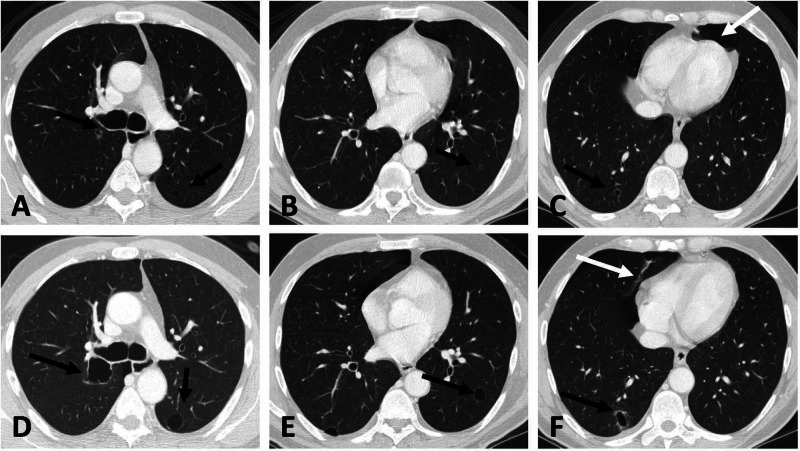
Enhanced CT at six-month follow-up (lung window) The thin wall cysts demonstrate enlargement in size exceeding 50% in volume and increase in wall thickness exceeding 3 mm in thickness, becoming cavitary in nature (images A, B, and C at three months; images D, E, and F at six months). Left pneumothorax completely resolved and a new pneumothorax developed on the right side (white arrow) CT: computed tomography
